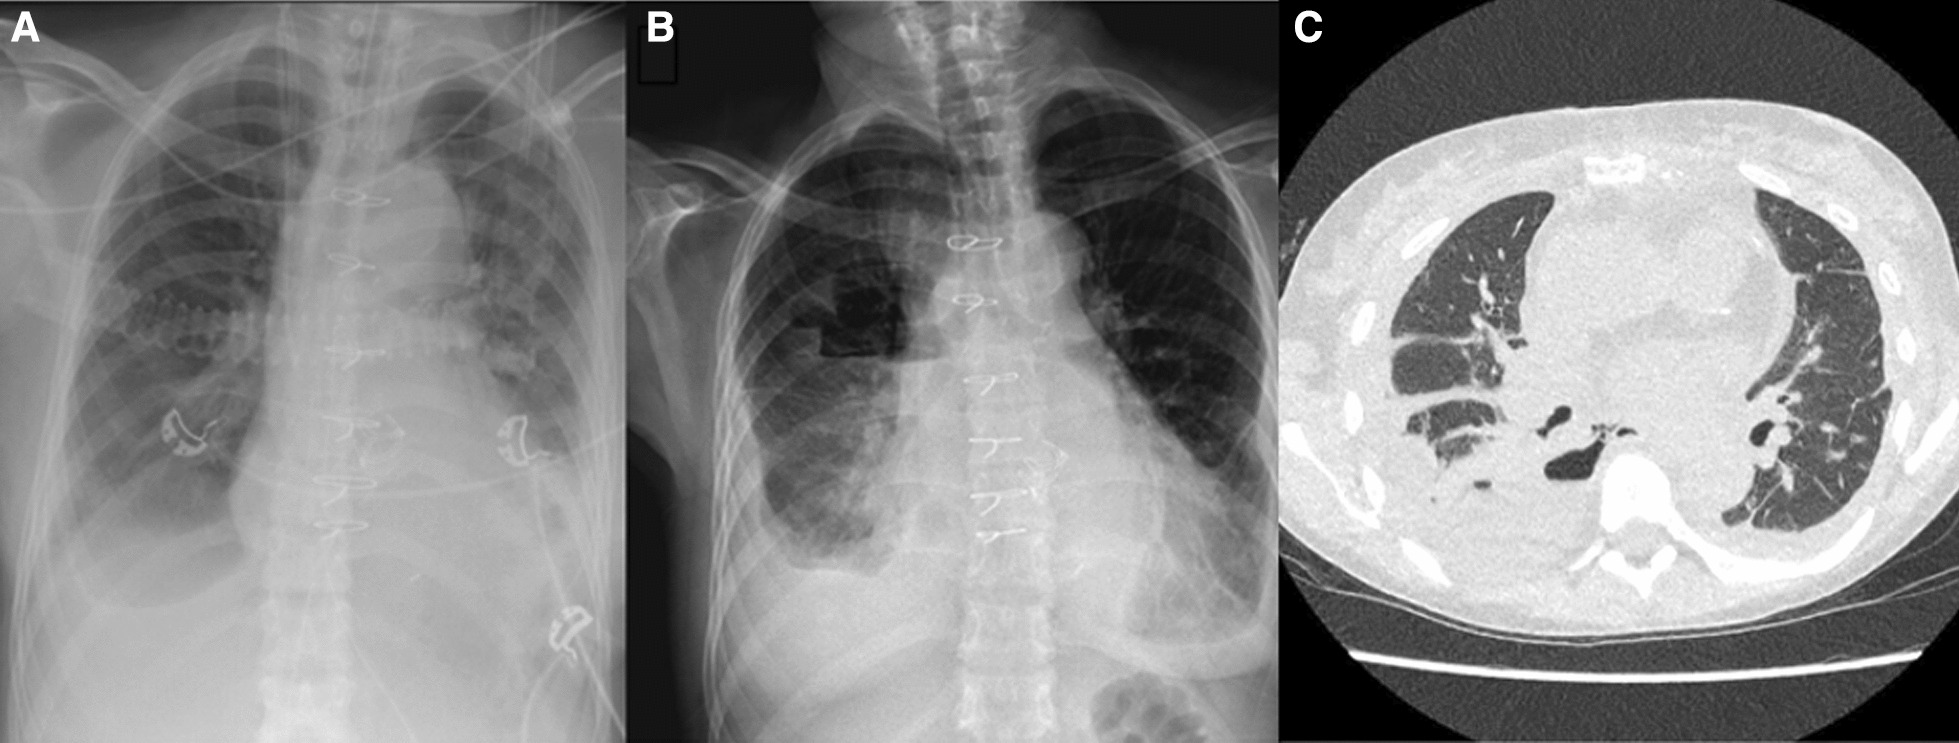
A postoperative chest X-ray on the ICU taken shortly after re-sternotomy because of the cardiac tamponade after AVR + CABG. B Regular control chest X-ray before discharge to referring hospital showing a new air-fluid level on the right side. C A coronal view of the CT-scan showing multiloculated air-containing pleural effusion strongly suspect of empyema
